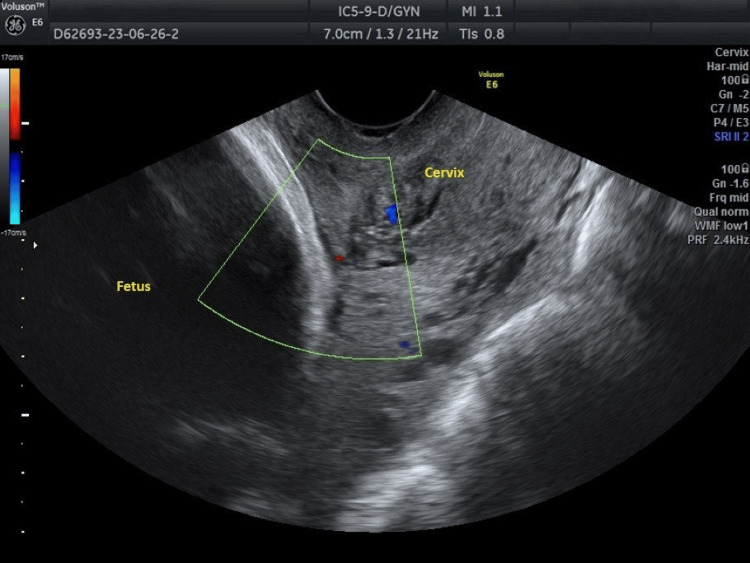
Transvaginal Doppler ultrasound imaging of the vasa previa during the third trimester of pregnancy Persistent blood flow near and around the internal cervical os further reinforces the suspicion of the presence of vasa previa.
